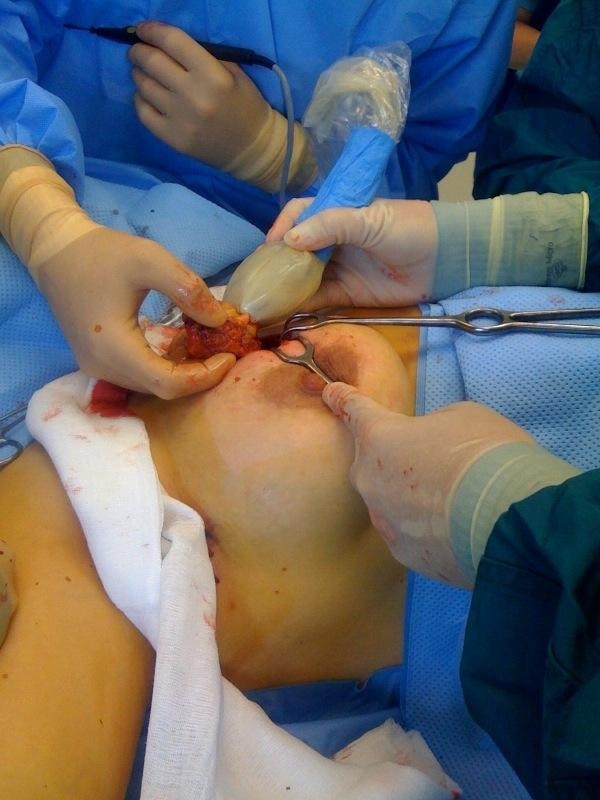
The ultrasound is applied repeatedly in the wound.
